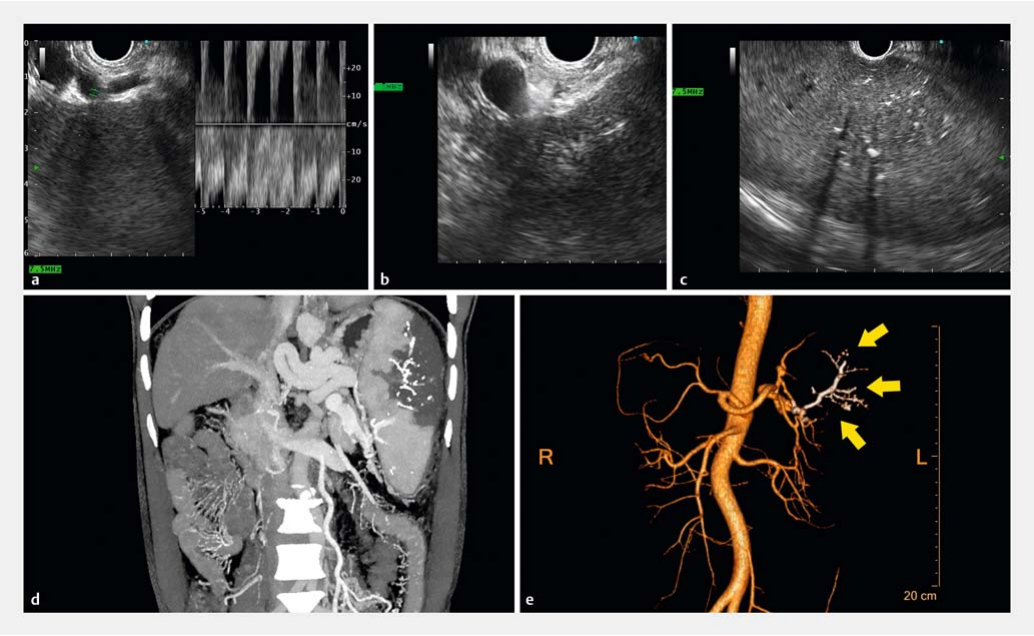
Endoscopic ultrasound (EUS)-guided super-selective partial splenic embolization.
